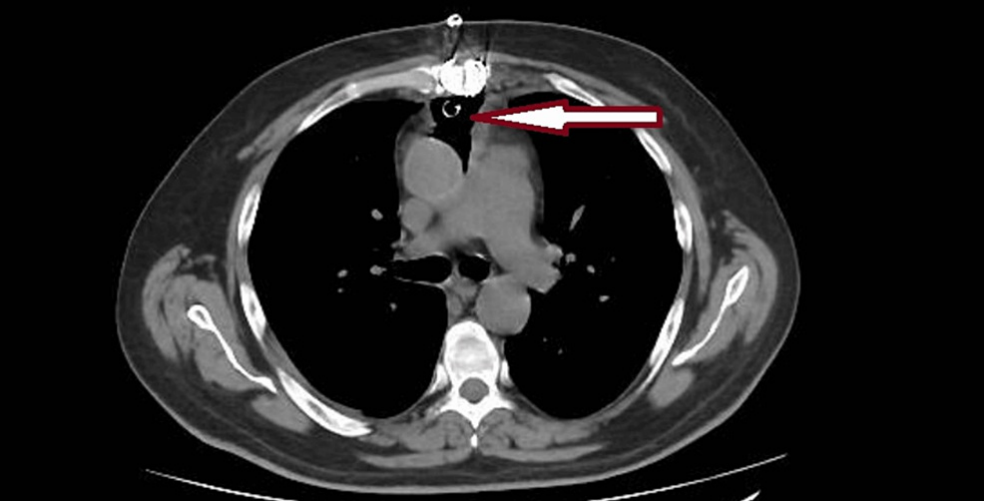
Postoperative chest CT demonstrating complete resolution of the loculated fluid from around the aorta (arrow)
